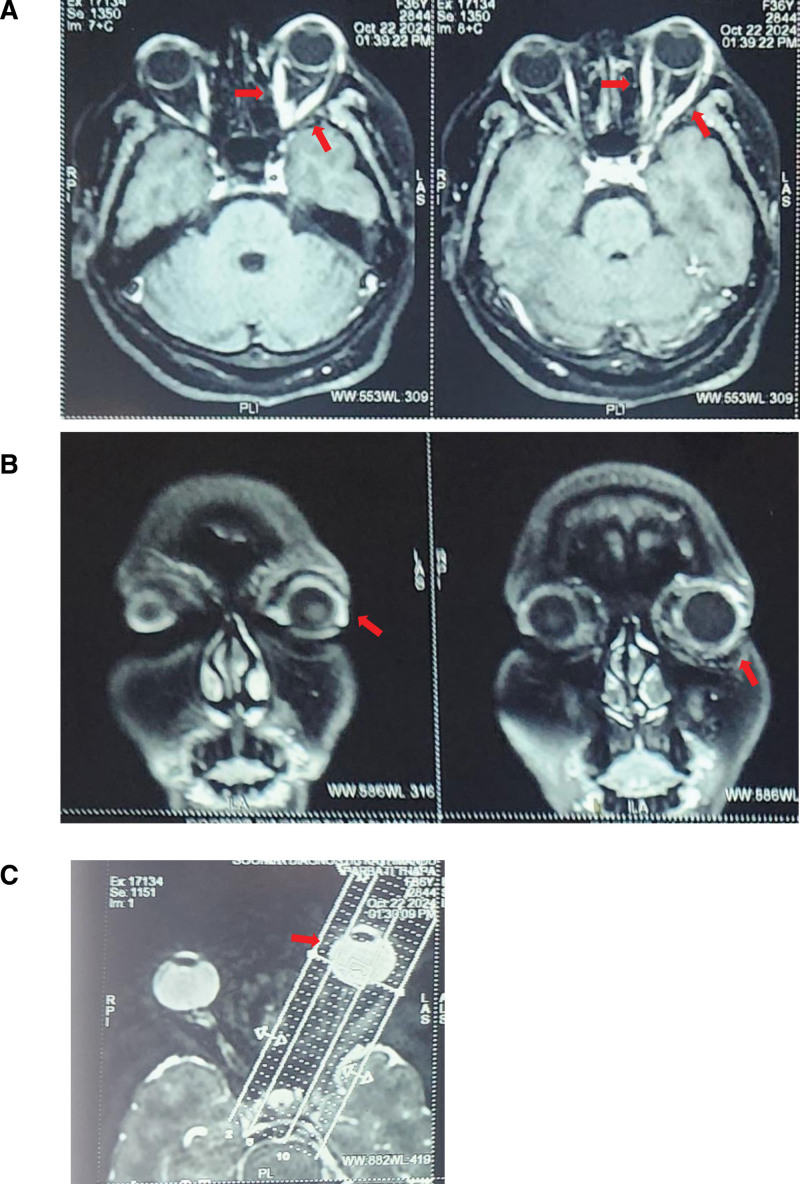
MRI of head and orbit (A and C, axial view; B, coronal view) showing proptosis of the left eye and thickening of the left extraocular muscles without the involvement of the tendons. MRI = magnetic resonance imaging.
